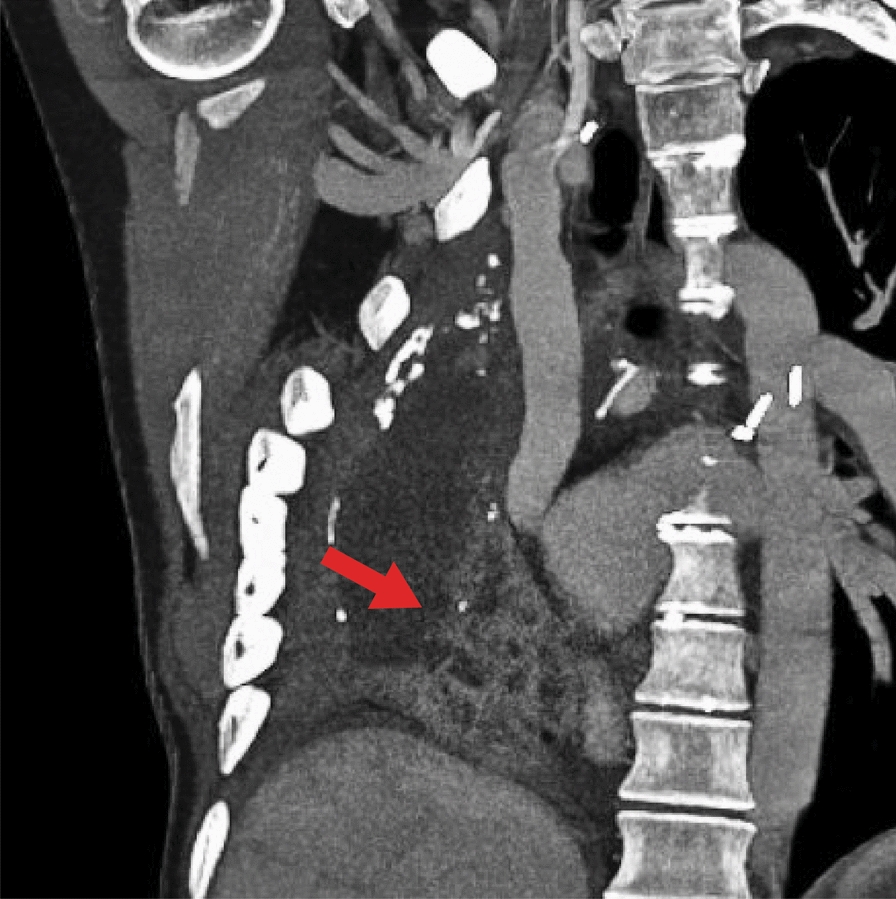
Chest CT scan showing mediastinal abscesses (arrow) between the pneumonectomy cavity, the heart and the diaphragm
